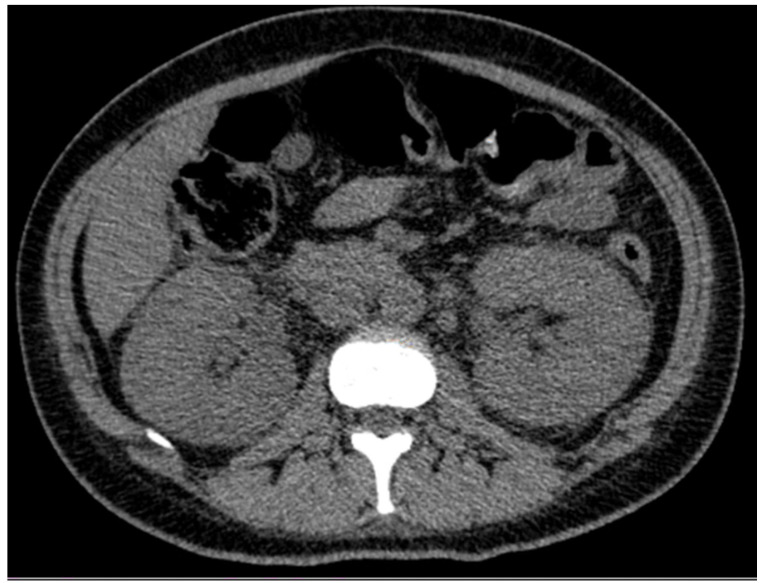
Abdominal and pelvic computed tomography scan revealing bilateral renal enlargement.
